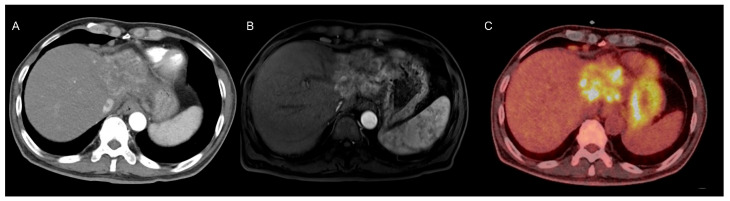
Multimodal Imaging of the Tumorous Lesion. (A) Computed tomography showing heterogeneous arterial enhancement within the lesion. (B) Gadoxetic-acid-enhanced liver magnetic resonance imaging of arterial phase demonstrating heterogeneous enhancement of the lesion. (C) Positron emission tomography–computed tomography of the liver demonstrating intense fluorodeoxyglucose uptake within the lesion.
